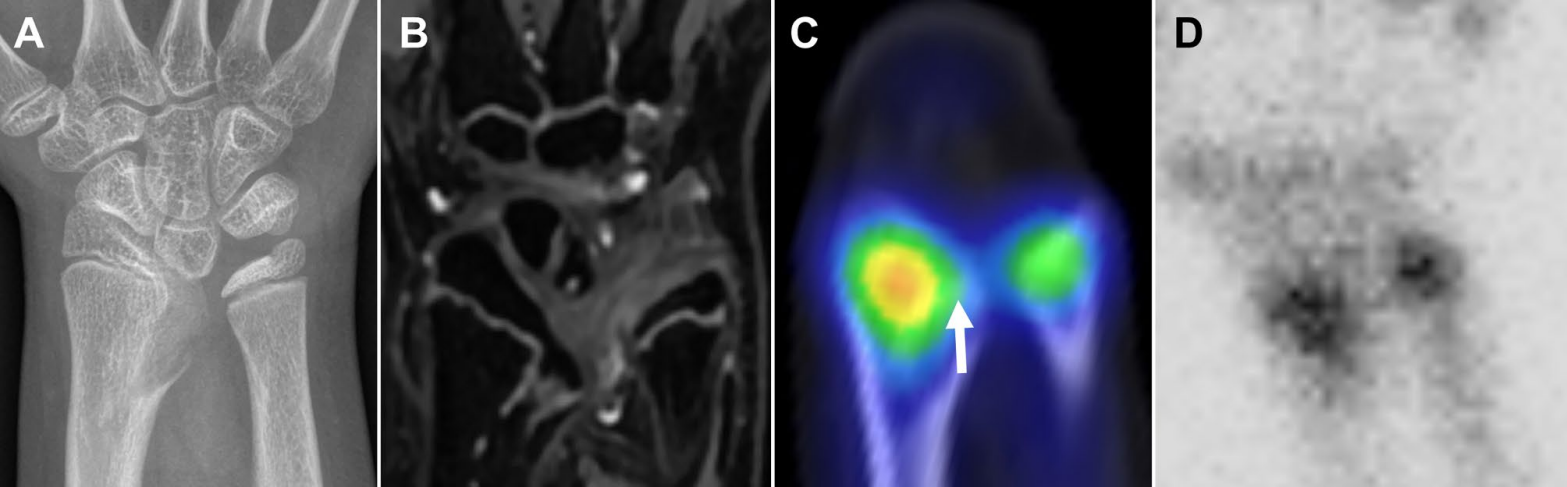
Advantage of bone SPECT/CT for evaluating growth disturbance of the small longitudinal bone. A 10.6-year-old girl with Leri-Weill dyschondrosteosis has Madelung’s deformity in both wrists (A). A physeal bar is not definitely identified on gradient-recalled-echo T2-weighted MRI (B). The ulnar side of the distal radial physis (arrow) is hypoactive compared with the radial side, suggesting that the deformity will aggravate over time (C). This discrepancy in physeal activity between the ulnar and radial sides is not evident on planar scintigraphy (D)
